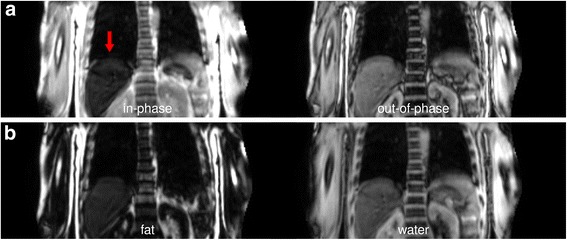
Coronal slice of in-phase and out-of-phase Dixon MR images (a) and determined fat and water images (b). The in-phase image demonstrates markedly low MR signals of the liver (arrow)
